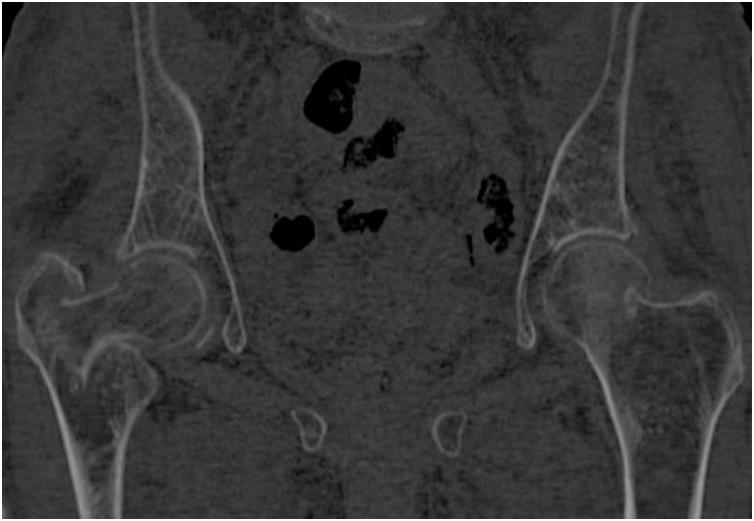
Preoperative CT scan of both hips confirmed the radiographic diagnosis and excluded pathological fractures.
